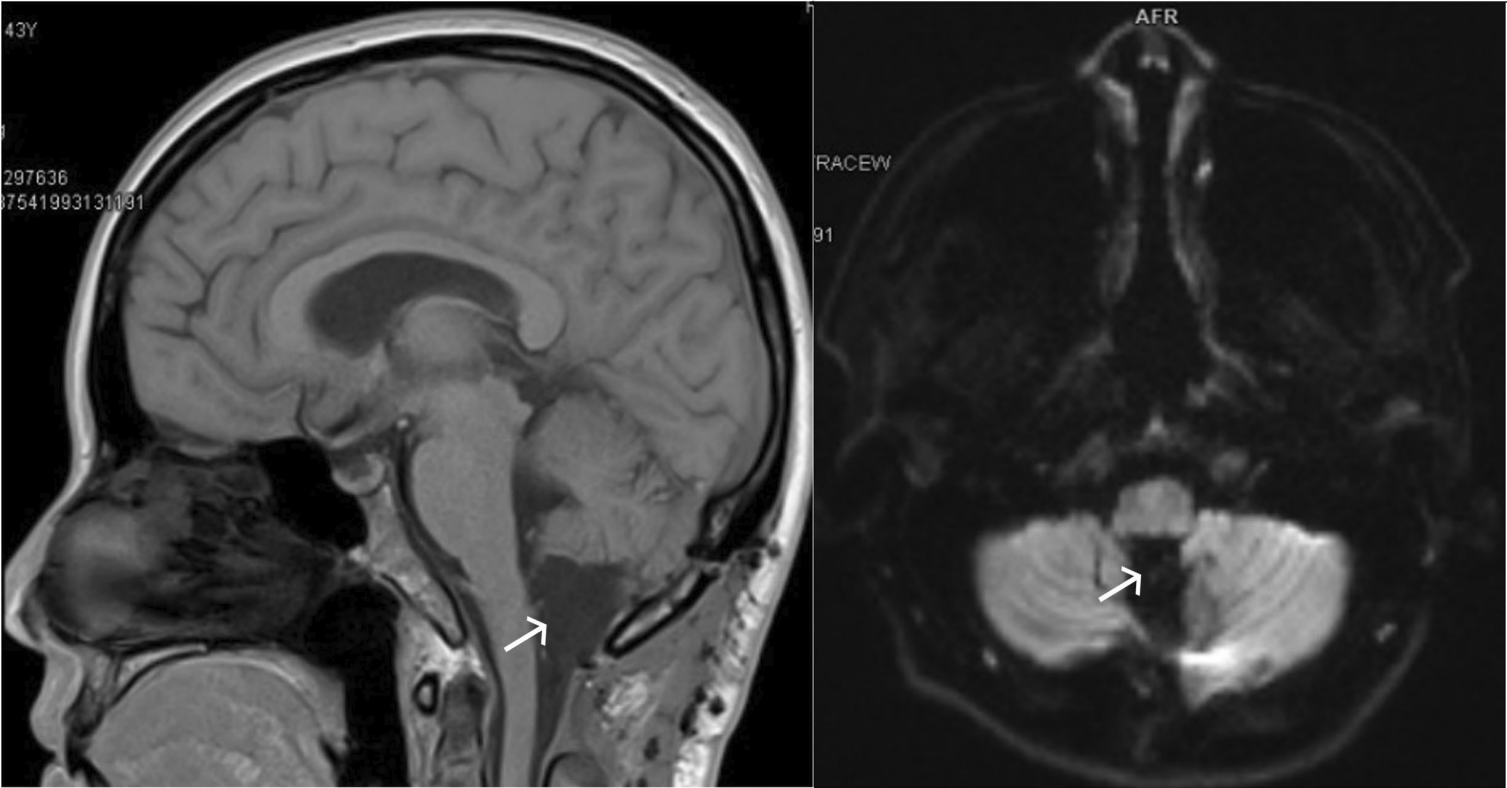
MRI of posterior fossa and cervical spine 1‐month post‐operation confirm no features of a cyst at the craniovertebral junction (white arrow).
